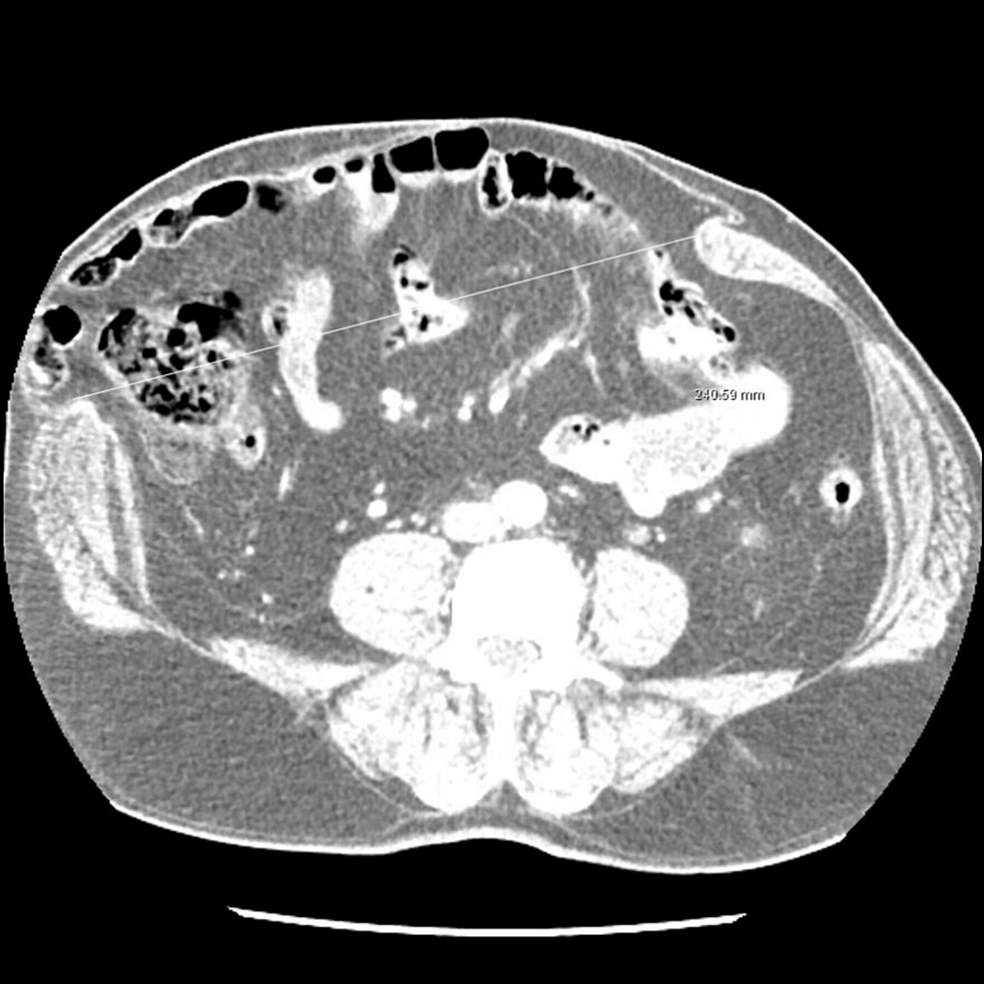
Axial section CT image of the sample patient with a fascial defect of approximately 24 cm
